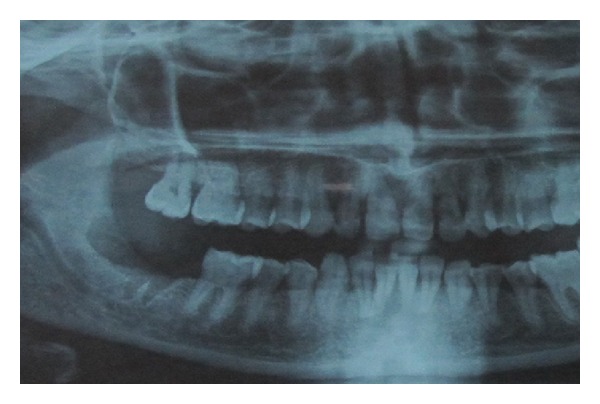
Radiographic appearance of persistent bony lucency in third molar and retromolar area after three months.
